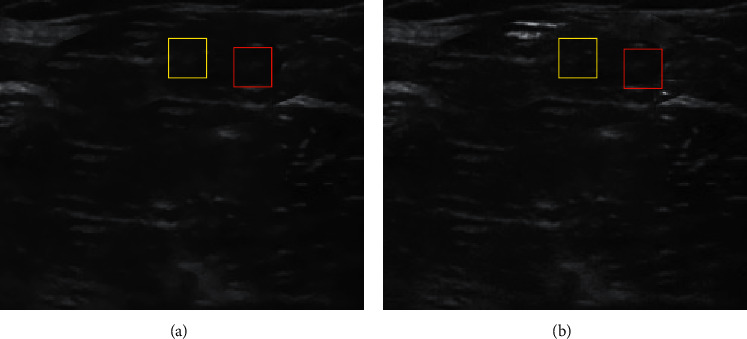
Ultrasound guidance based on blind deblurring algorithm. (a) was the initial ultrasound-guided imaging, and (b) was the ultrasound-guided imaging after blind deblurring algorithm optimization.
